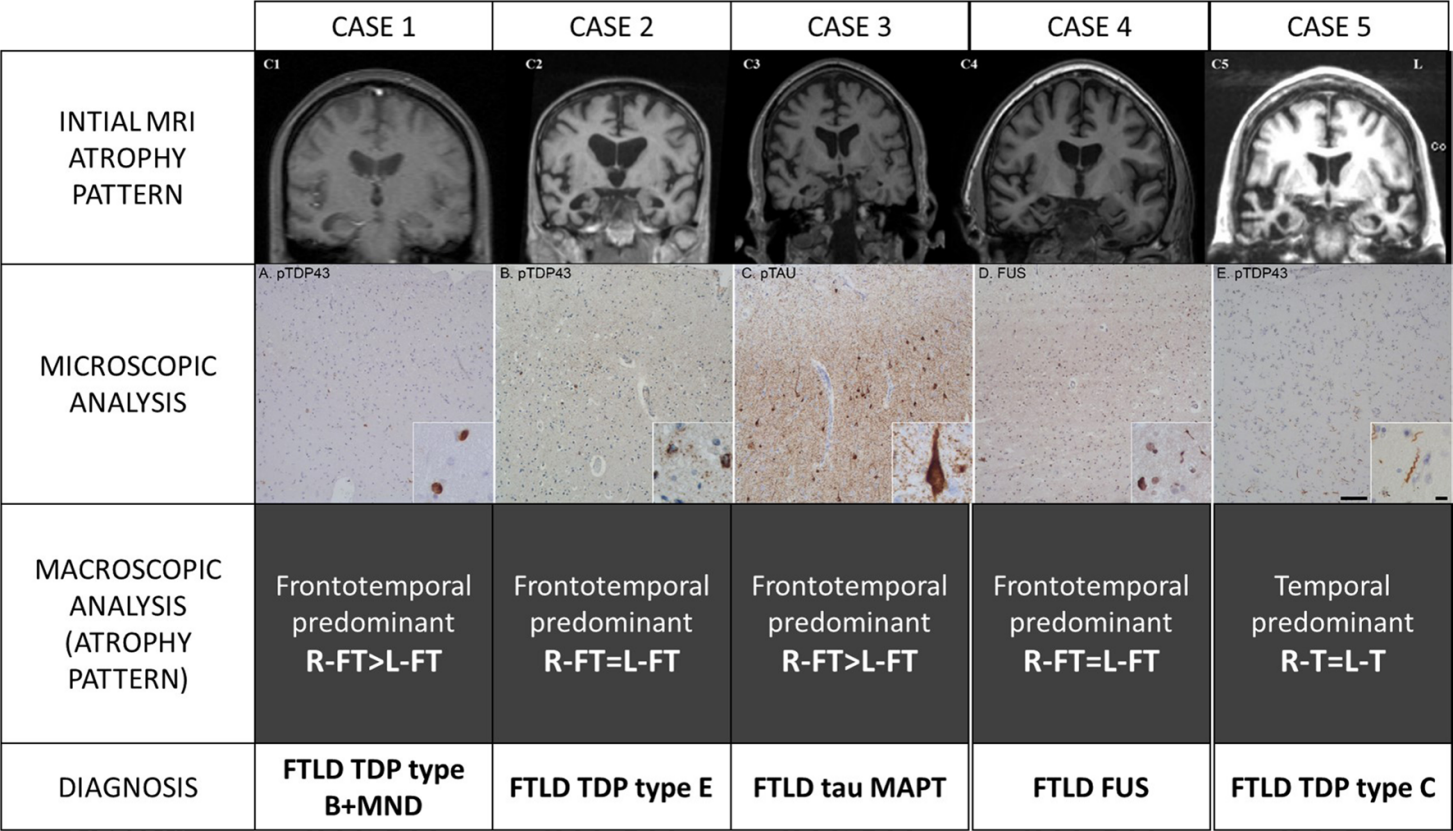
Different pathological diagnoses in donors with rtvFTD. The cases with rtvFTD displayed pathology from different pathological molecular subclasses in FTD. Although all pathological accumulations started from the right temporal lobe, according to the initial MRI atrophy pattern, over time the patients exhibited heterogeneous progression patterns. Case 1 showed FTD-TDP-B pathology with predominant neuronal inclusions throughout the cortical layers in right temporal lobe (a). Clinically, motor neuron disease developed over the disease course. Case 2 showed FTD-TDP-E pathology characterised by granulofilamentous neuronal inclusions (insert) and grains in right temporal lobe (b). The pathology spread to bilateral fronto-temporal areas. Clinically, this was accompanied by severe behavioural and language problems. Case 3 had tau-pathology with threads and tangles and some plaques (Anterior cingulate cortex: C, adapted from Ulugut Erkoyun et al.,2021, JAD, CC BY-NC 4.0). At the end stage of the disease, right predominant frontotemporal atrophy was observed based on the macroscopic pathological examination. The clinical evolution involved the development of atypical Parkinsonism. Case 4 had large FUS-positive neuronal inclusions and FUS-positive threads (D: right frontal lobe), developed severe global atrophy at a clinical picture of becoming mutistic and bedridden in 4 years after diagnosis. Lastly, case 5 showed long dystrophic neurites characteristic for FTD-TDP-C (E: insular cortex) and developed bilateral temporal atrophy at the end stage of the disease, based on the pathological examination. This patient’s clinical features were relatively benign, presenting with verbal and non-verbal semantic impairment and without the development of any motor disturbances and a disease duration of 12 years. Scalebar is 100 μm, scalebar insert is 10 μm
